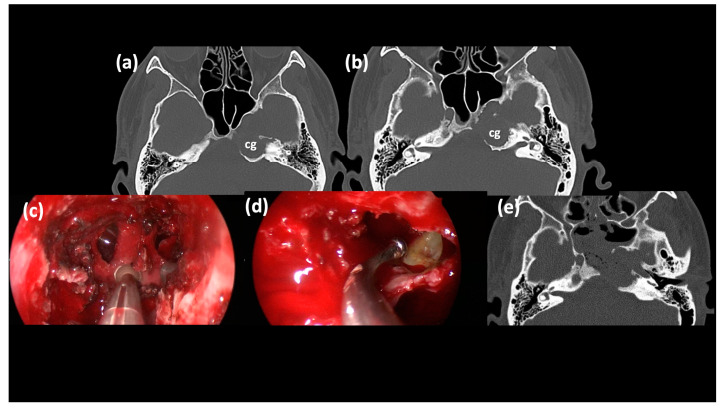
Trans-sphenoidal approach. (a,b) Axial CT scan sections of a left PACG. (c) Surgical step: with a diamond bur the sphenoid sinus is opened. (d) Surgical step: with a curve dissection tool the cyst of the PACG is opened and drained. (e) Post-surgery CT scan with regular trans-sphenoidal drainage. cg, cholesterol granuloma.
